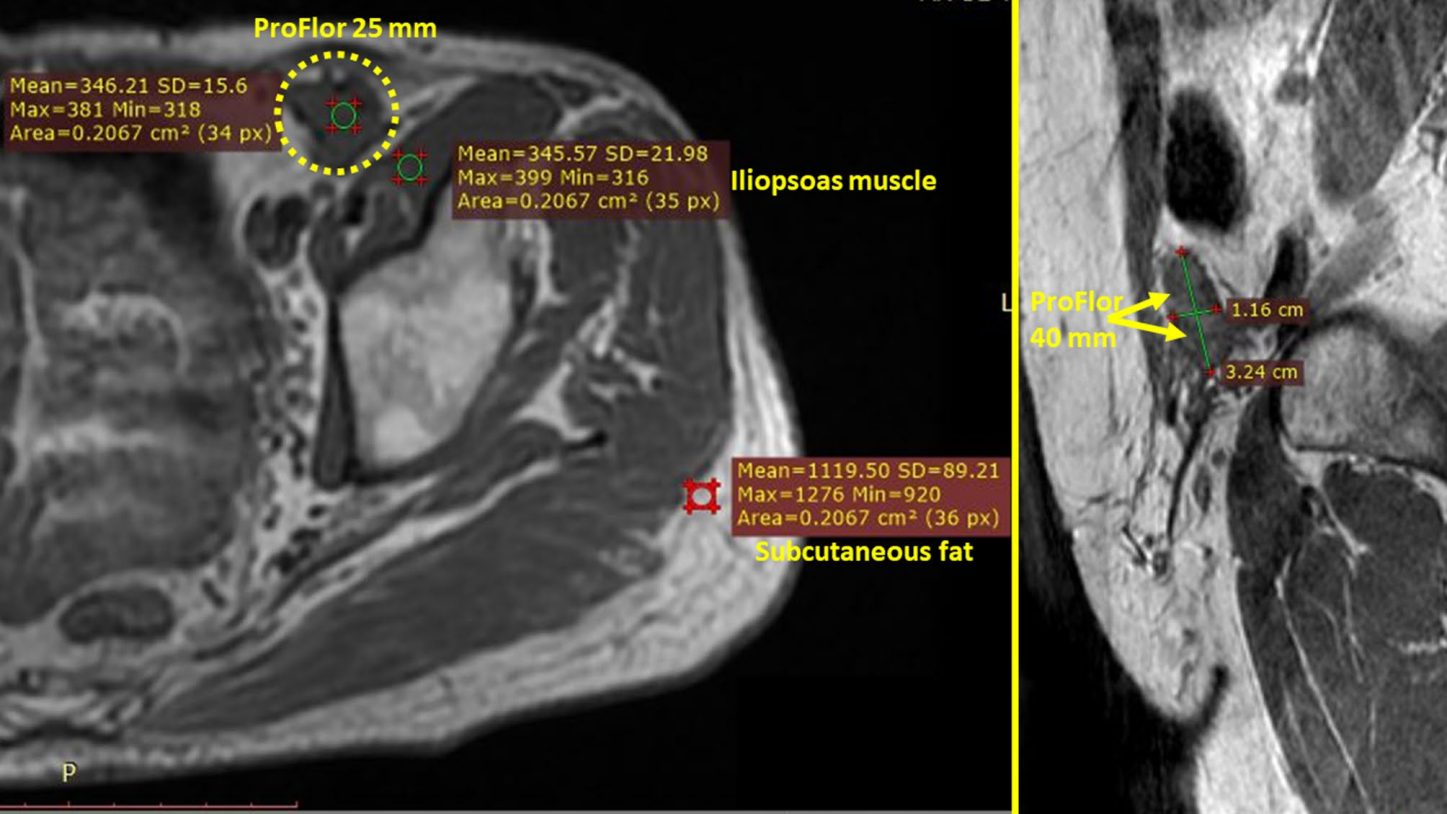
(A) Axial T1 SE MR image of the pelvis. (A) Patient with left inguinal hernia defect repaired with 25 mm ProFlor 9 months after implantation. 3D scaffold (yellow circle) occupies former hernia defect in the groin and appears wholly integrated into the inguinal backwall. 3D structure appears fully incorporated by newly ingrown tissue. The mean signal intensity (SI) of the newly developed tissue within ProFlor is nearly identical to the SI of the neighboring left iliopsoas muscle. Subcutaneous fat tissue of the lateral abdominal wall shows significantly higher SI. (B) Detail of sagittal T1 SE MR image of the pelvis showing a 40 mm ProFlor 12 months after implantation. 3D hernia device (yellow arrows) fully obliterates the defect. Transverse thickness of 3D scaffold, originally 15 mm, under steady pressure of visceral content, is reduced to approx. 11 mm, which is comparable to the thickness of the muscular barrier of the groin. Diameter of ProFlor, originally 40 mm, being squeezed within the former hernia defect now measures 32 mm.
